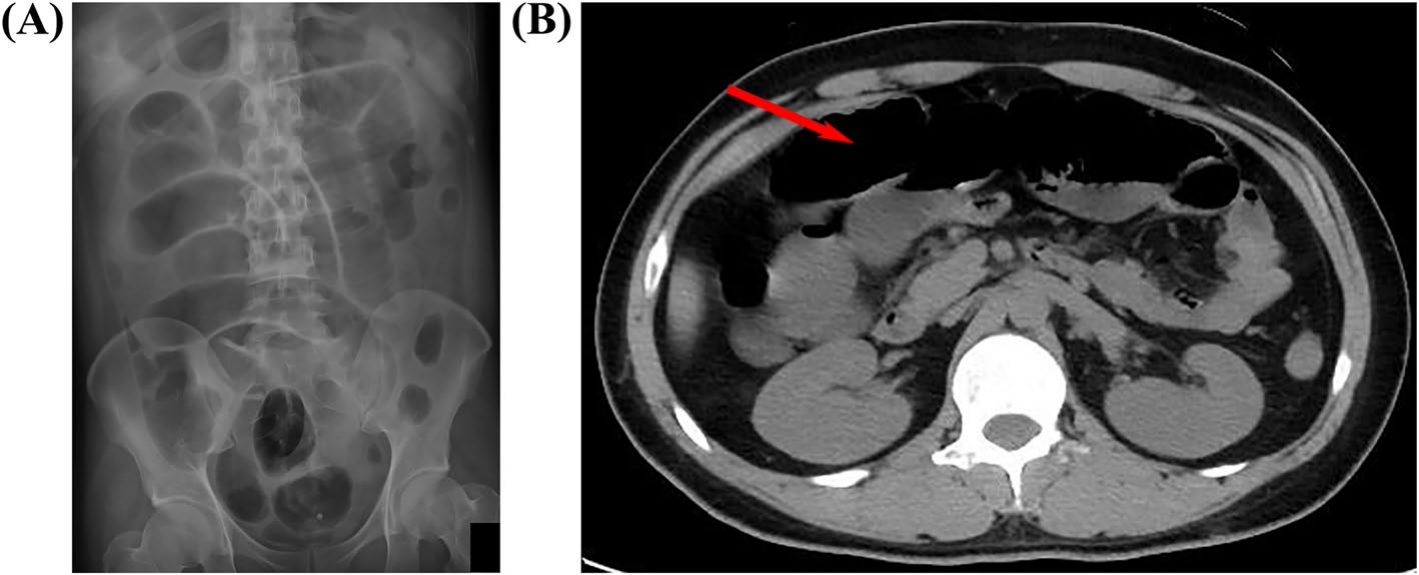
Abdominal radiograph and CT on admission. A Abdominal radiograph revealed intestinal gas and dilatation of the intestine.B Abdominal CT showed dilatation (red arrow) and fluid retention in the small intestine
